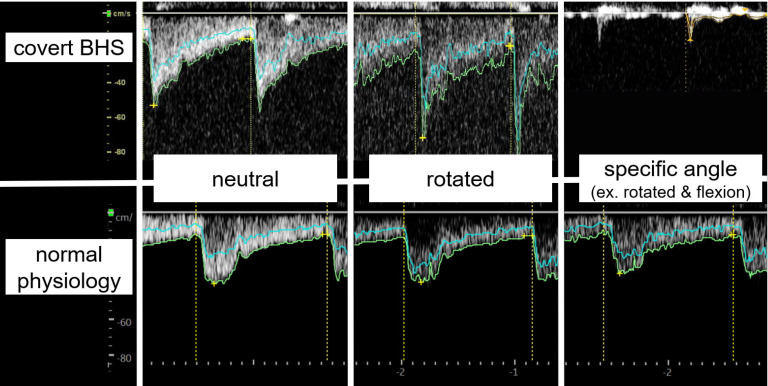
Doppler ultrasound findings of covert BHS and normal physiology. In normal physiology, there is no decrease in the EDV at any cervical angle. In covert BHS, however, there is an apparent decrease in the EDV only at specific angles. However, covert BHS shows a typical waveform only by merely rotating the neck horizontally, which is its main difference from common BHS. Abbreviations: EDV, end-diastolic flow velocity; BHS, bow hunter's syndrome.
